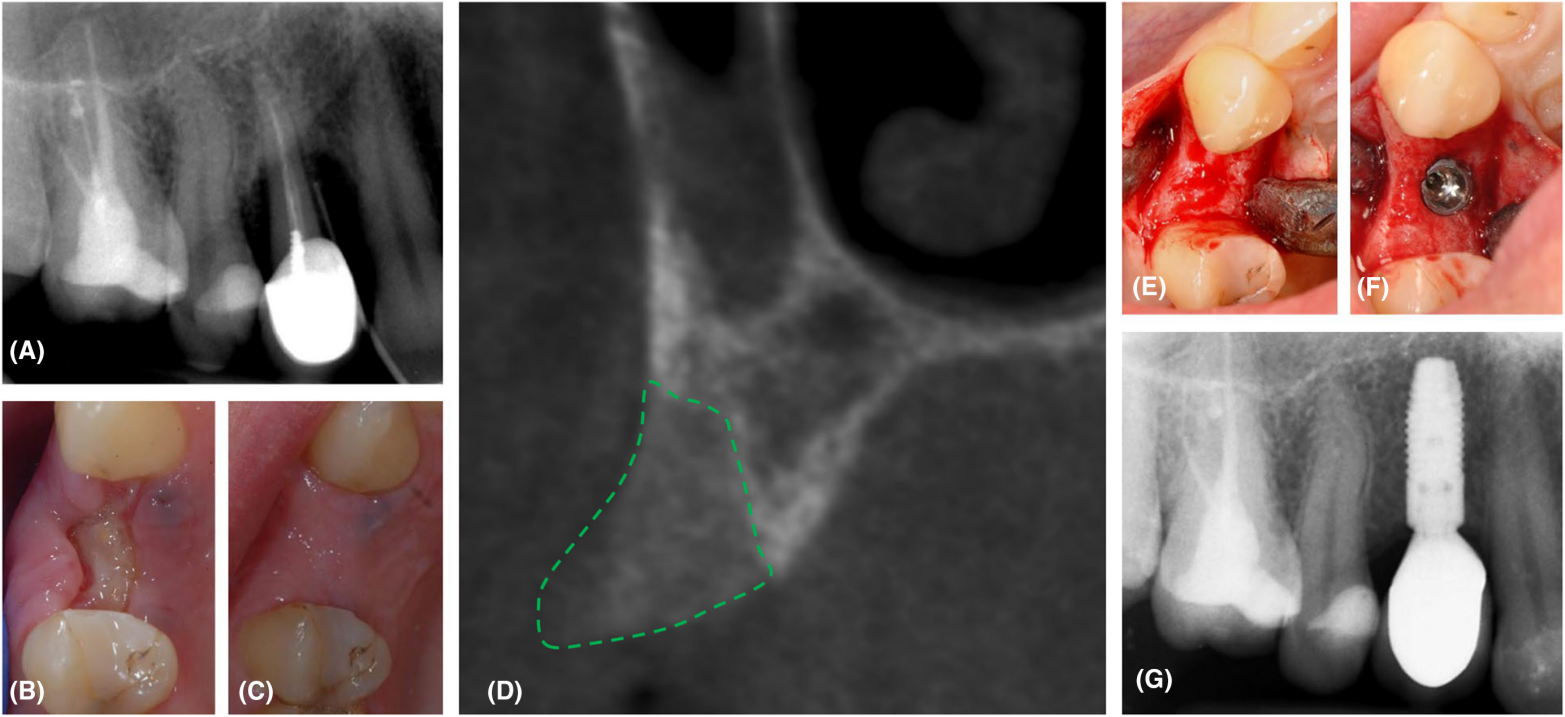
Clinical case of ARP with L‐PRF in the posterior zone. (A) The first premolar in the upper right side was extracted (loss of the entire buccal bone plate, PPD > 10 mm, endodontic pathology); (B) After 1 week, the soft tissue starts covering the top L‐PRF membrane, which is sealing the entrance to the socket; this membrane shows some pink spots (probably due to vascularization); (C) Good soft tissue healing after 3 months with minimal change in alveolar crest dimensions; (D) Cross‐sectional CBCT image in the center of the extraction socket showing optimal bone regeneration with moderate bone calcification; (E) Re‐entry after 3 months with the entire re‐generation of the buccal bone plate; (F) Insertion of a 3.6 mm diameter Astra‐Tech EV implant; (G) Intra‐oral radiograph after 7 years of loading.
